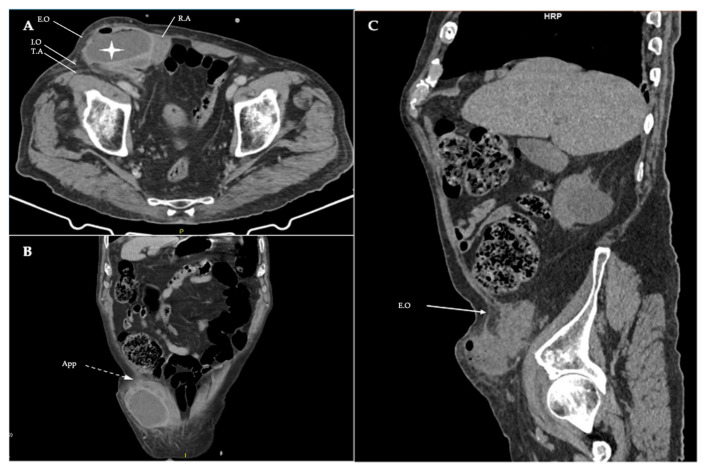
(A) Axial view of contrast-enhanced CT showing the organized abscess of the abdominal wall (white star) and the fascia defect of the Spigelian hernia, as well as the rectus abdominis (R.A), the right-side external oblique aponeurosis (intact—E.O), the internal oblique (I.O) and transversus abdominis (T.A). (B) Coronal view, highlighting the appendix (dashed arrow—App) protruding through the fascia defect. (C) Sagittal view, in which the muscle layers of the abdominal wall and the hernia orifice are best identified, with external oblique aponeurosis remaining intact (solid arrow—E.O).
